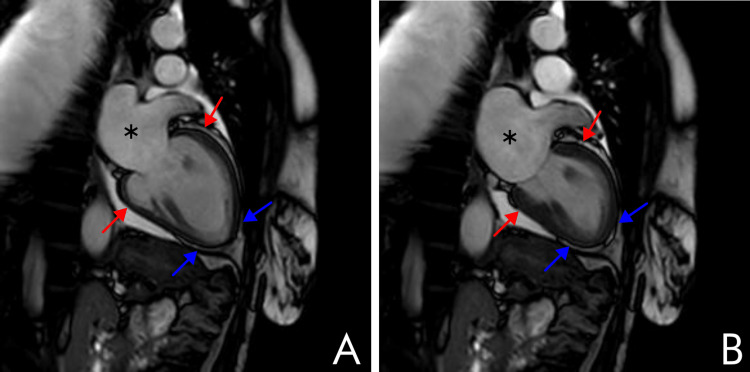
Cardiac MRI showing biventricular takotsubo cardiomyopathy T2 sequence of a cardiac MRI in sagittal view showing two moments of the cardiac cycle, diastole (A) and systole (B), respectively. The study reveals a hyperkinetic apical third (red arrows) with an akinetic basal third (blue arrows). Additionally, there is severe left atrial dilation (black asterisks), alongside a mild pericardial effusion in lateral and inferior walls. No late enhancement was reported. These findings suggest biventricular takotsubo cardiomyopathy and effectively rule out inflammatory myocarditis. MRI: magnetic resonance imaging
